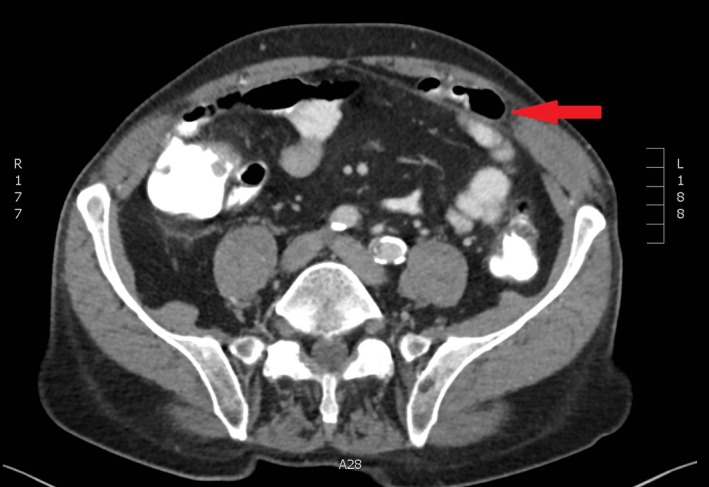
Transversal image of CT scan showing incarcerated herniation in the lower left quadrant of the abdomen (arrow)
